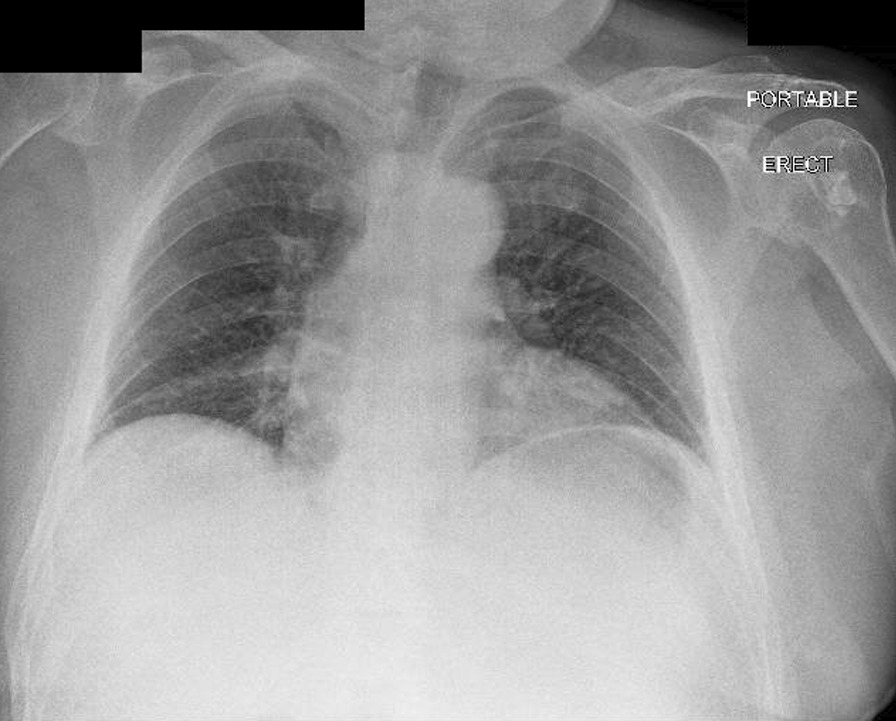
Portable X-ray of chest done in emergency department
